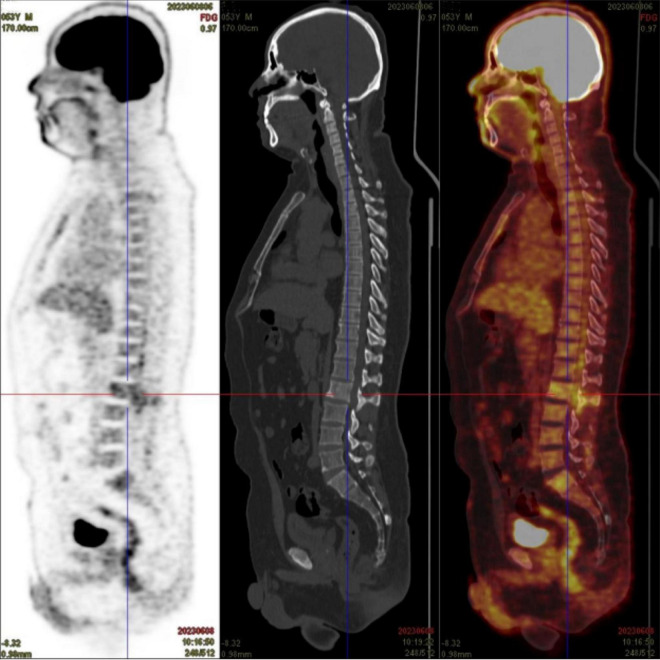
The PET/CT scan of the patient showed areas of uneven density in several vertebrae. Slightly increased FDG uptake was observed in the vertebral bodies and appendages, with active FDG metabolism also detected in the spinal cord cavity between the T12 and L2 segments.
